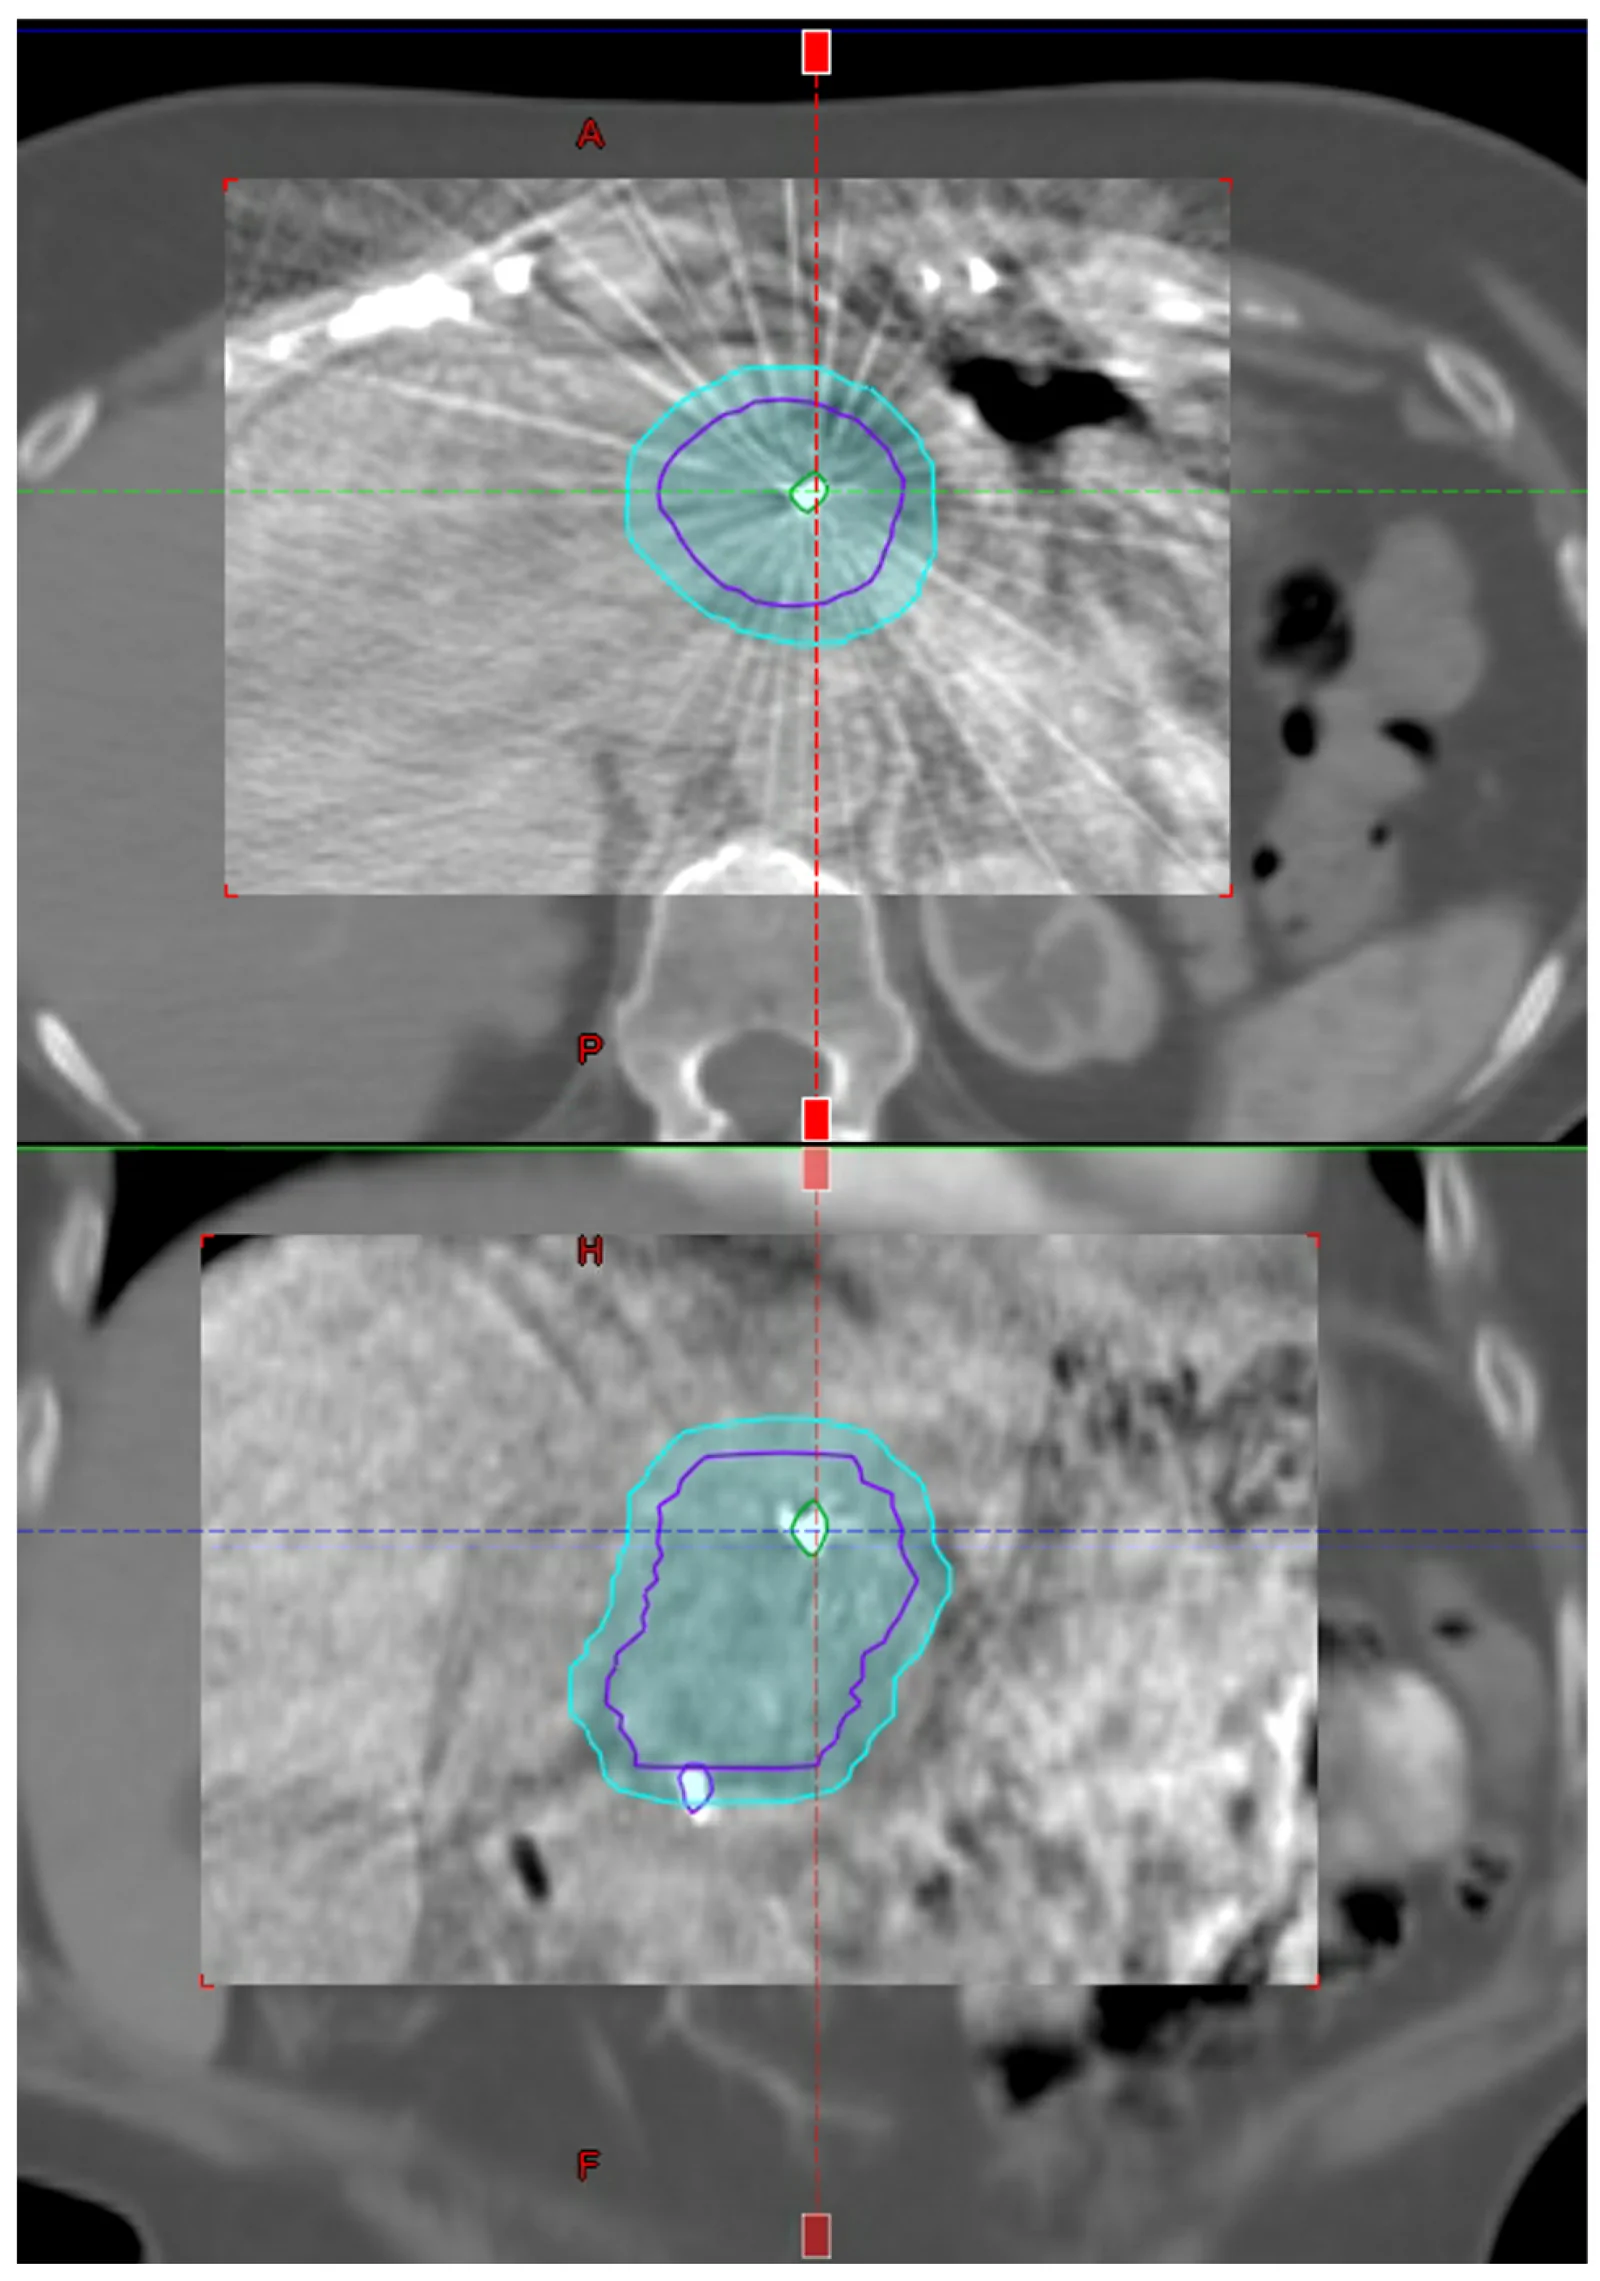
Cone beam CT of a patient with match of fiducials. The targets are ITV (shaded area in violet) and PTV (cyan). The green contour is a fiducial seed.
